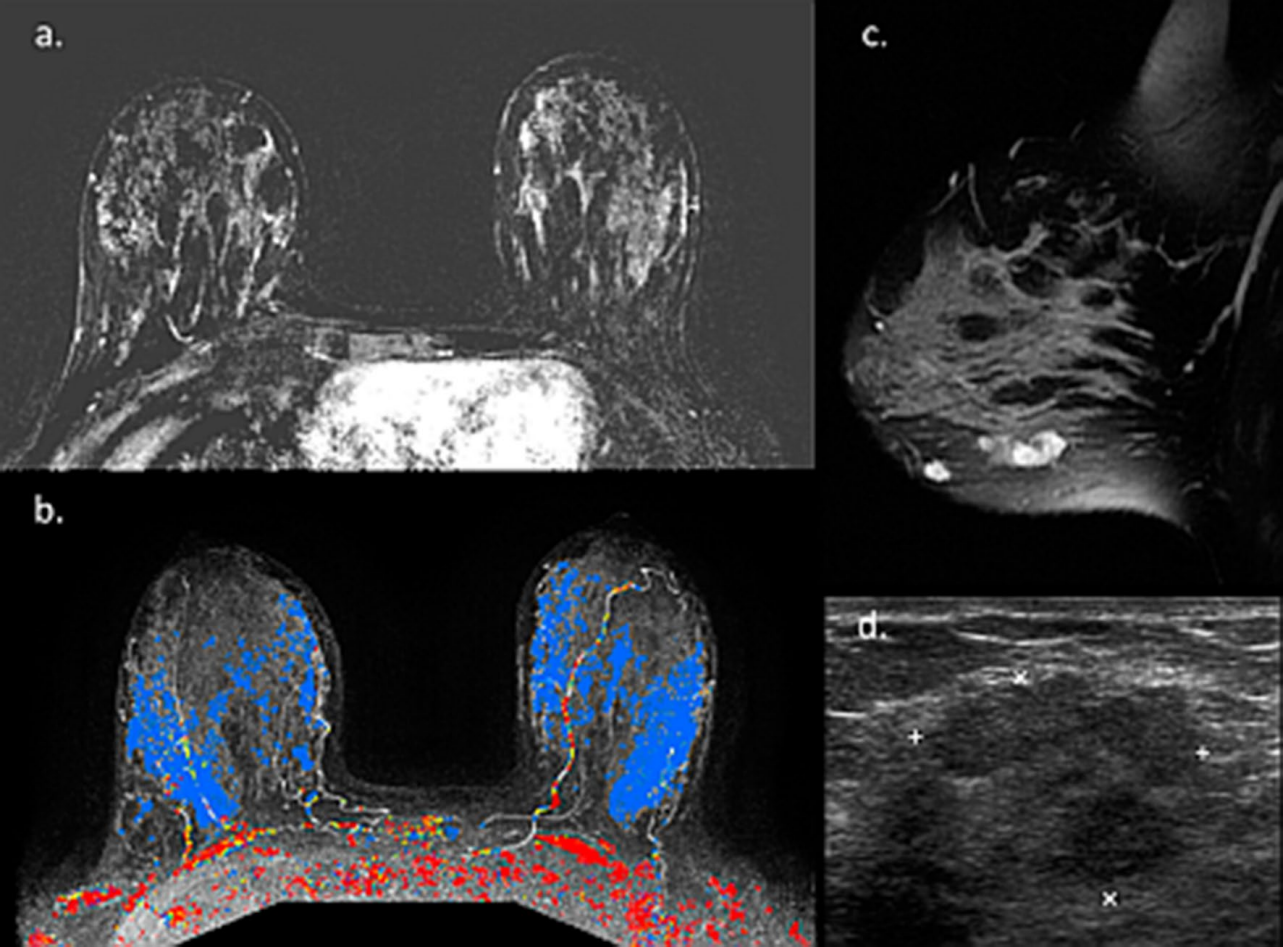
MRI of mucinous carcinoma during pregnancy. MR images of patient with left sided mucinous carcinoma, presented as a palpable mass during pregnancy are presented. The patient chose to undergo elected abortion. Subtraction DCE image reveals marked bilateral BPE (a) and CAD reveal bilateral diffuse benign-like coloring with progressive enhancement in time intensity curves (b) without evidence of the known underlying lesions in the left breast. However, the lesions, diagnosed on US (d), were clearly visible on sagittal T2-weighted fat-suppressed image (c)
